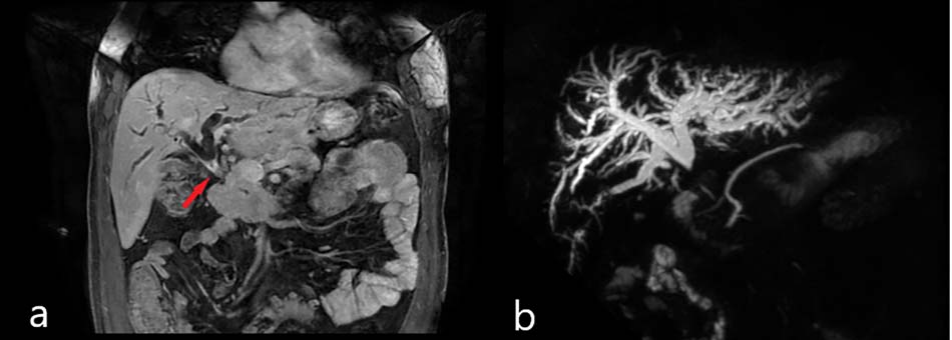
Magnetic resonance imaging (MRI): (a) coronal scan showing the dilatation of bile ducts and the thickening of the MBD (red arrow); (b) magnetic resonance cholangiopancreatography (MRCP) showing the dilatation of intrahepatic bile ducts and the absence of signal from the MBD below the confluence to the papilla of Vater.
